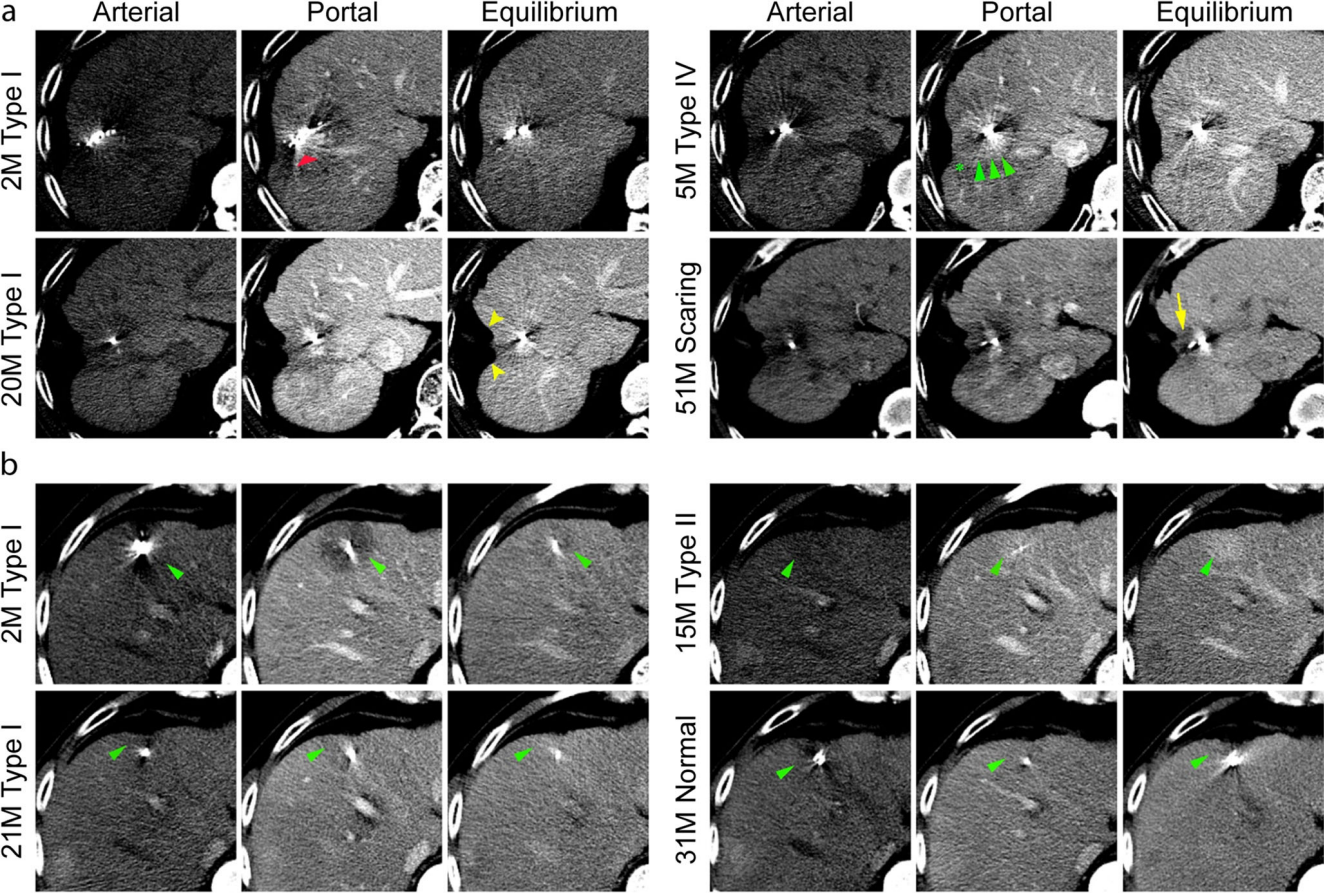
Typical conversion and consequence. a The initial reaction was type I with several artifacts (red arrow) in a 54-year-old man with hepatocellular carcinoma. The reaction converted to type IV at 5 months with a low-density band (green star) surrounding a high density (green arrow). At 20 months, the reaction appeared as type I again, with local atrophy (yellow arrow). At 51 months, scarring around the seeds was observed, showing a low density (yellow arrow). b CT images at 2, 15, and 21 months post-implantation demonstrated a mutual conversion between types I and II (green arrow) in a 58-year-old man with hepatocellular carcinoma. The reaction recovered to normal at 31 months
